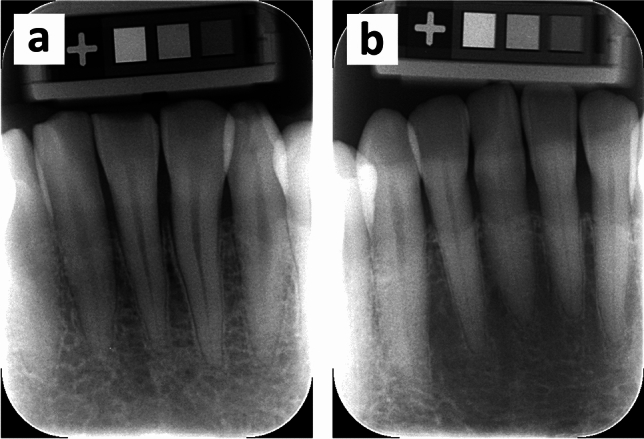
Two representative radiographic images demonstrating the highest (a: 1259.26 mg/cm^2^, male, 28 years) and lowest (b: 495.24 mg/cm^2^, female, 78 years) al-BMD values in the analysis. Dense trabeculation (a) and sparse trabeculation pattern (b) are predominant. Marginal bone loss is commonly less than two-thirds of the root length.
